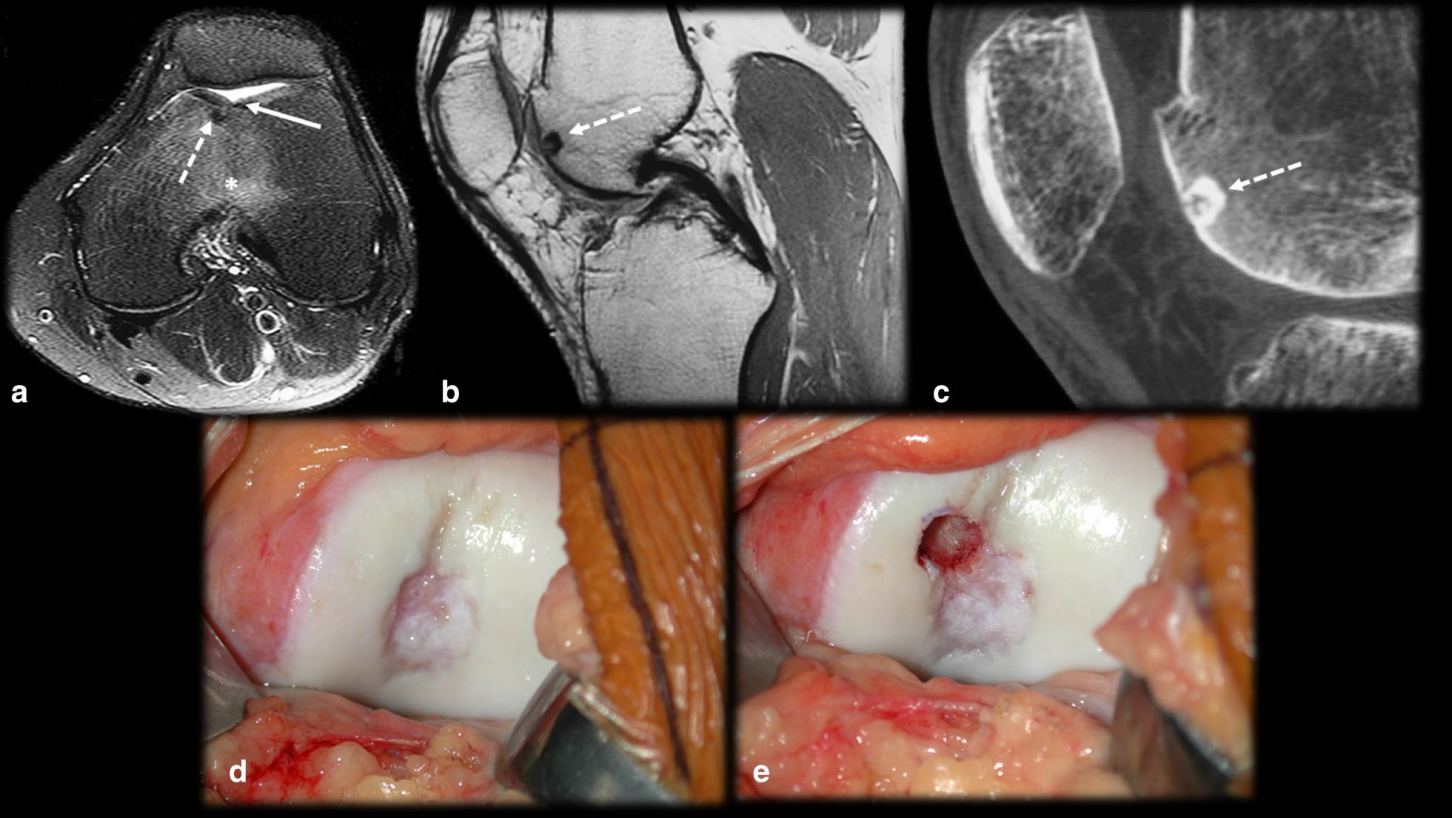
Subchondral osteoid osteoma mimicking trochlear chondromalacia. A 35-year-old male with anterior knee pain for 4 months. Axial T2 FS MR images (a) showed deep chondral erosion (arrow), subchondral edema (asterisk) and a small, low signal intensity foci that could be a nidus (dashed arrow). Sagittal T1 MRI (b) and CT (c) confirmed the diagnosis of the OO nidus (dashed arrow). Surgical images before (d) and after (e) resection
